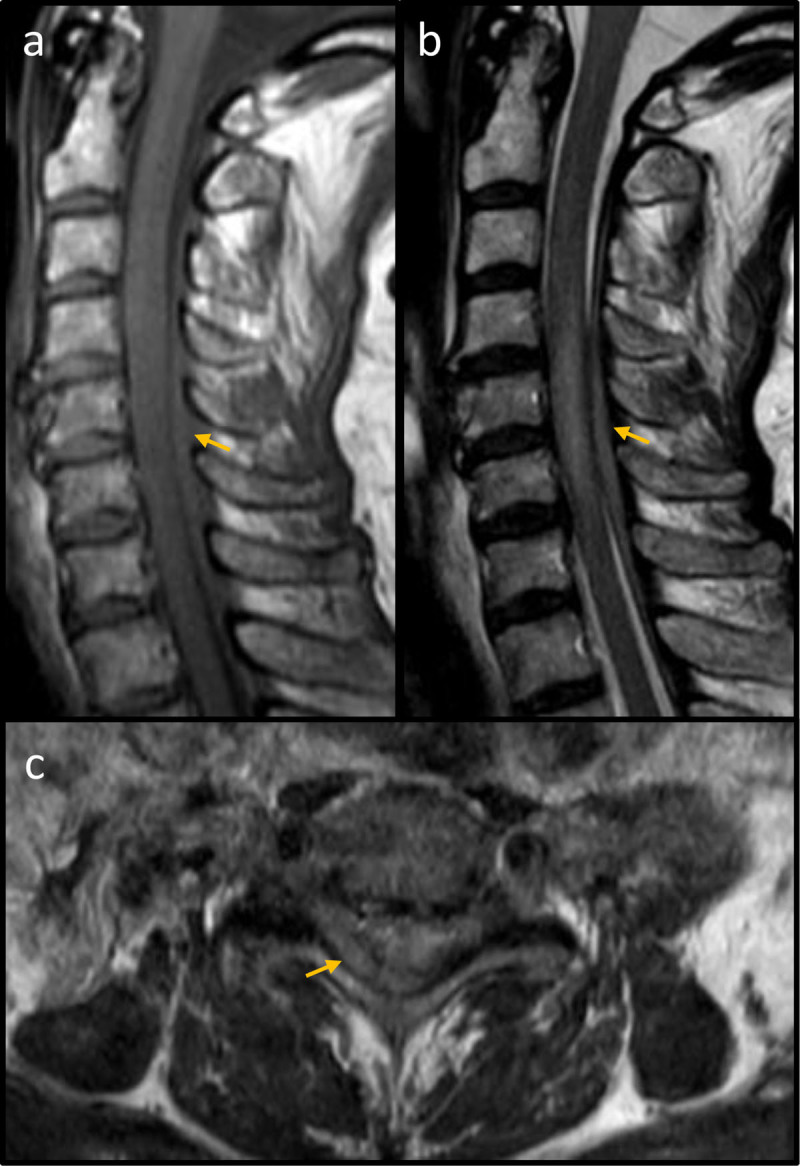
MRI of the cervical spine. (a) Sagittal T1 spin echo demonstrating an iso-intense space-occupying lesion (yellow arrow) restricted to the posterior epidural space. (b) Sagittal T2 spin echo showing a slightly hyper-intense mass (yellow arrow) in the posterior epidural space with corresponding myelopathy. (c) Axial T2 spin echo at vertebral level C6 showing a predominantly right-sided mass in the posterior epidural space (yellow arrow).
